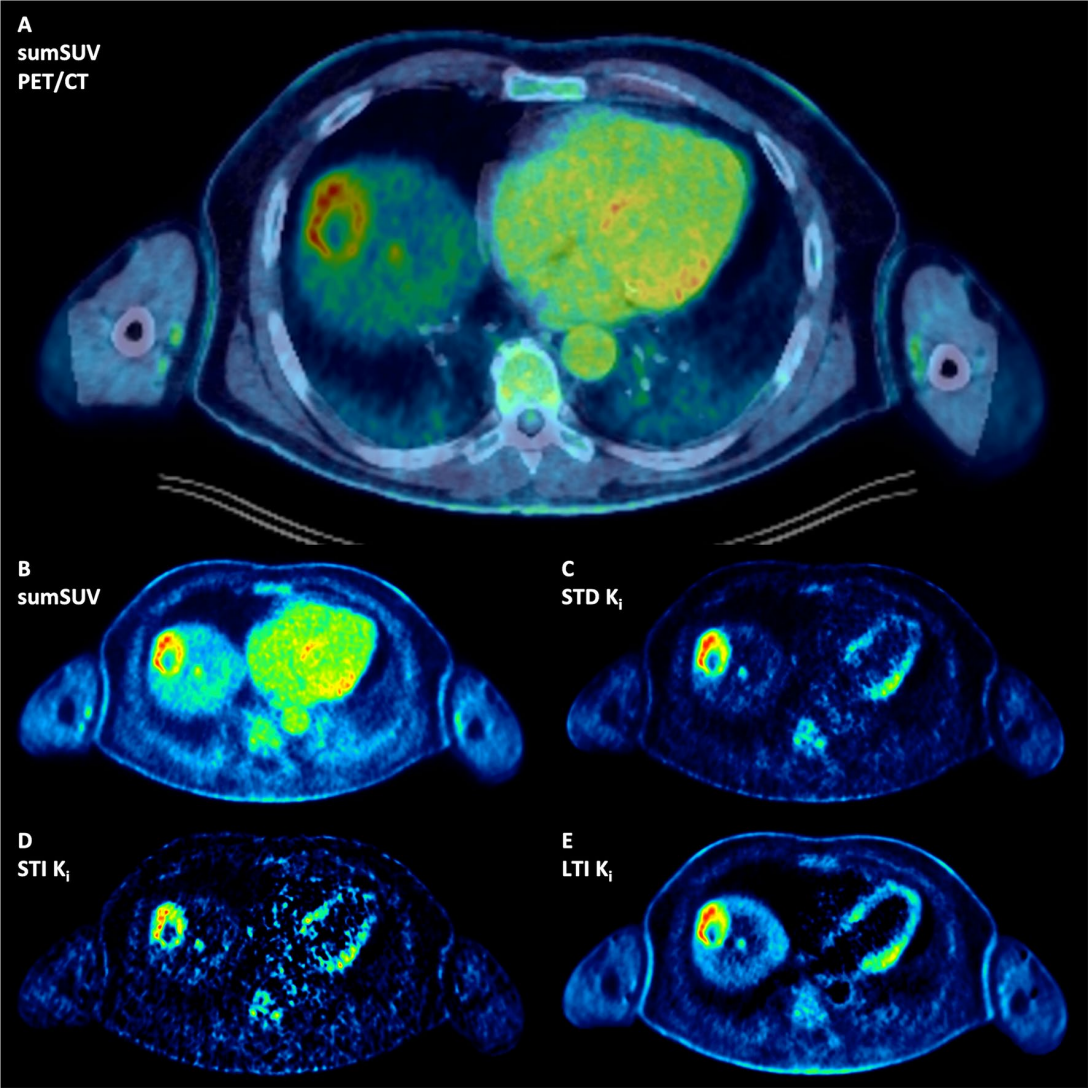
Patient with multiple liver metastases from uveal melanoma: All Patlak K_i_ images demonstrate a small liver metastasis in segment 8 which is not clearly delineated in the sumSUV (A fused PET-CT and B) SUV images due to high background of the liver. The STD Patlak K_i_ image (C) demonstrates better contrast and less background than LTI Patlak K_i_ image (E) and less noise than STI Patlak k_i_ image (D)
